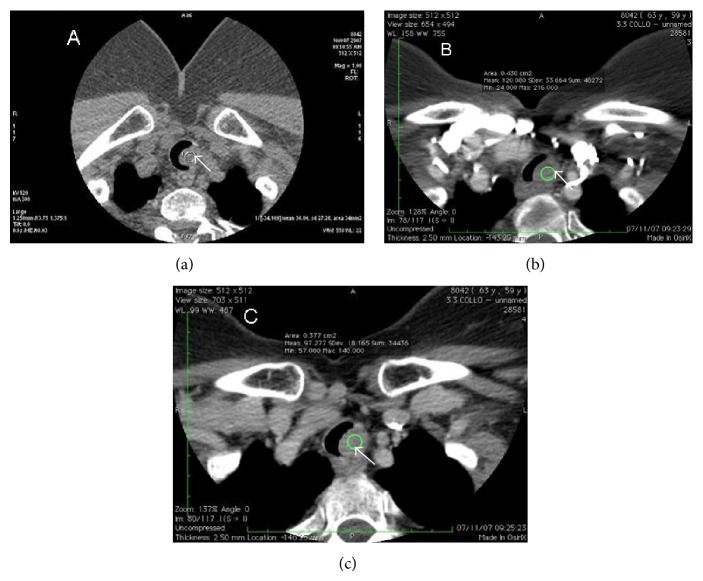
64-year-old female with granular cell tumor of trachea. Axial multiphase contrast-enhanced CT (16-slice Multidetector CT, GE Medical Systems) demonstrating polypoid lesion (diameter 13 mm) (white arrows), which presents smooth and well-defined margins and occupies the left side of the tracheal lumen. This mass does not present infiltrative signs suggestive of malignancy. The lesion shows elevated contrast enhancement in arterial phase and a modest release of contrast in venous phase; this behaviour is the tomographic expression of the rich vascularity of this kind of tumor. (a) Basal: 36 HU. (b) Arterial phase: 120 HU. (c) Venous phase: 100 HU.
